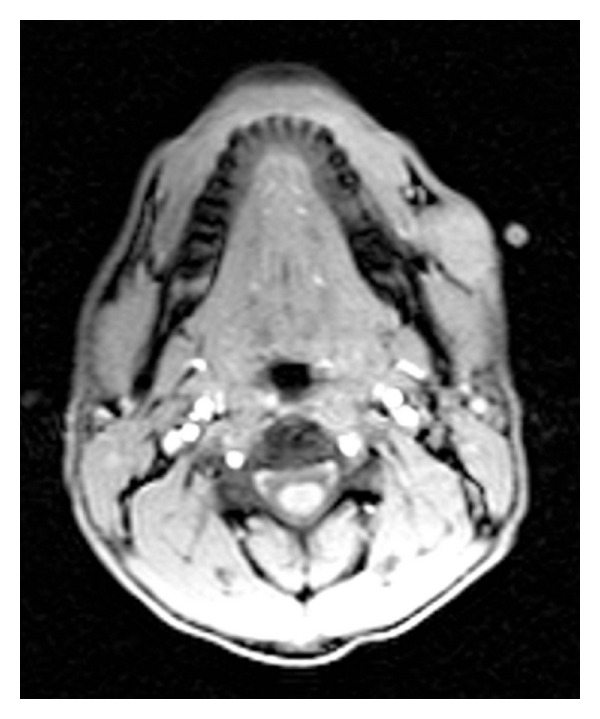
Axial view of a T2 magnetic resonance image at the level of the mandible showing a heterogeneous mass on the left side of the face with no evidence of deep invasion.
